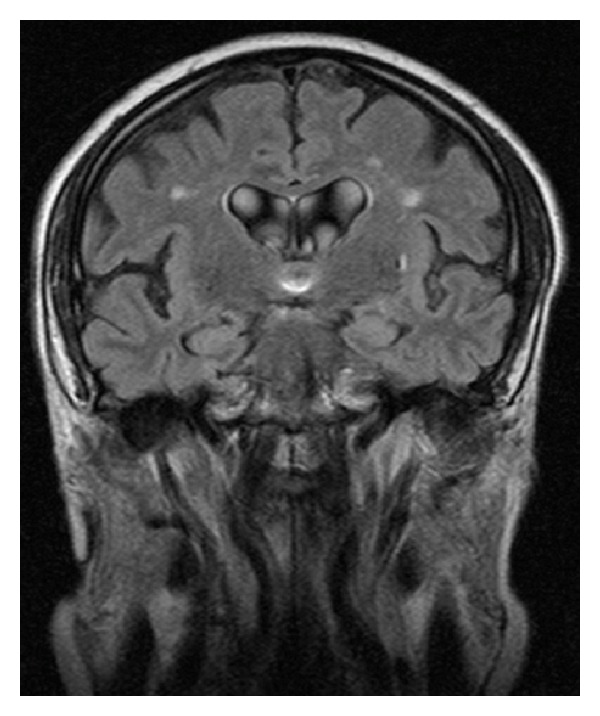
MRI Flair—multiple subcortical biparietal lacunar infarctions.
